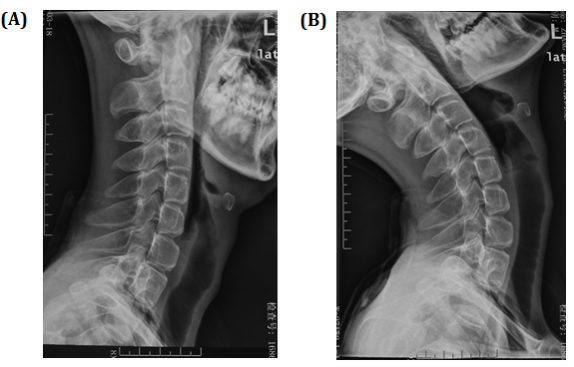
X-ray of cervical flexion and extension
